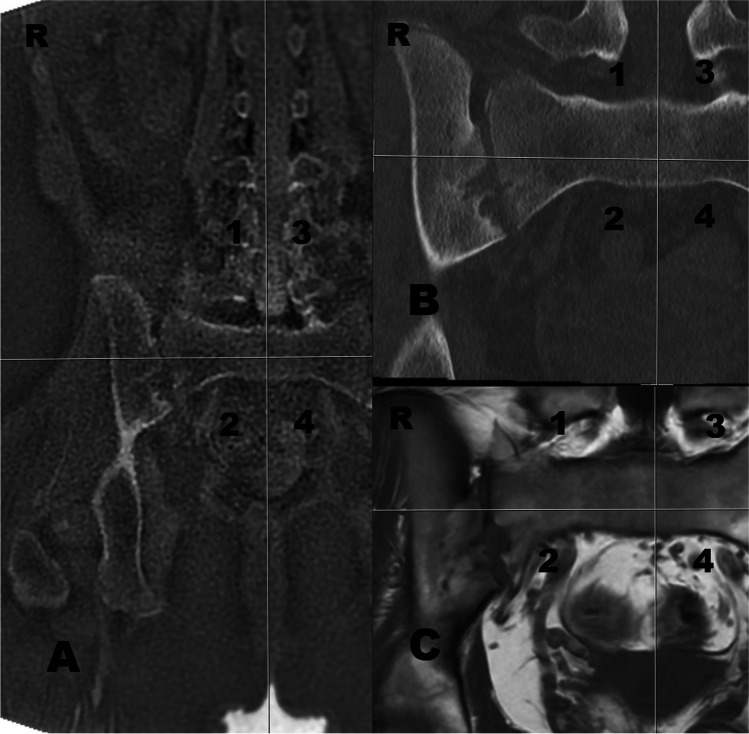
A 16-year-old girl with sacroiliitis. a Coronal ZTE-MRI received a score of 0 in the 1st segment and a score of 2 (pseudowidening) in the 2nd segment. b Coronal low-dose CT received a score of 0 in the 1st segment and a score of 2 (pseudowidening) in the 2nd segment. c Coronal T1-weighted image received a score of 0 in the 1st segment and a score of 2 (pseudowidening) in the 2nd segment. *CT*, computed tomography; *ZTE-MRI*, zero–time echo magnetic resonance imaging
